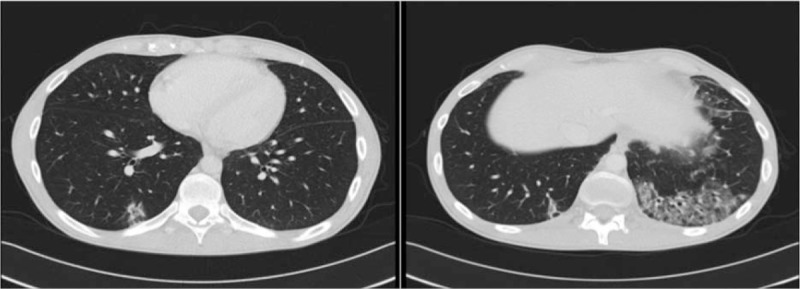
Chest HRCT of patient #3. Representative images of patient #3's thoracic HRCT at the time of PAH diagnosis, showing ground glass opacities mainly located in the left inferior lobe, and occupying less than 20% of total lung parenchyma. HRCT = high-resolution computed tomography, PAH = pulmonary arterial hypertension.
